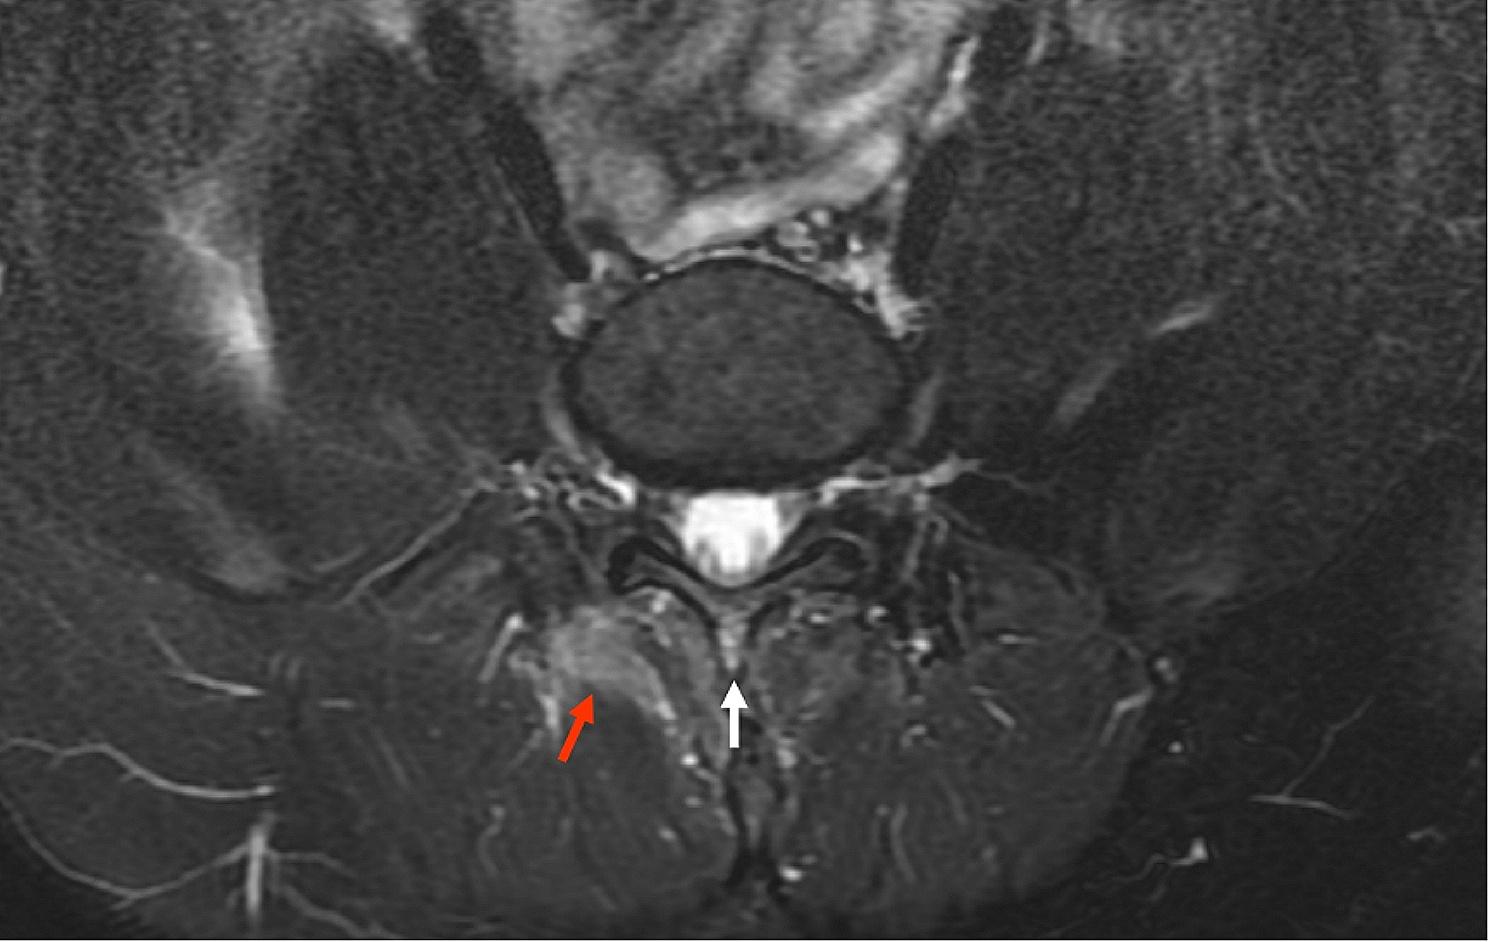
Enthesitis in a 19-year-old male with axial spondyloarthritis. Transverse MR image exhibited enthesitis at the spinous process of the L5 vertebra, visualized as bone marrow edema at the spinous process (white arrow) and inflammation at the adjacent soft tissue (red arrow)
